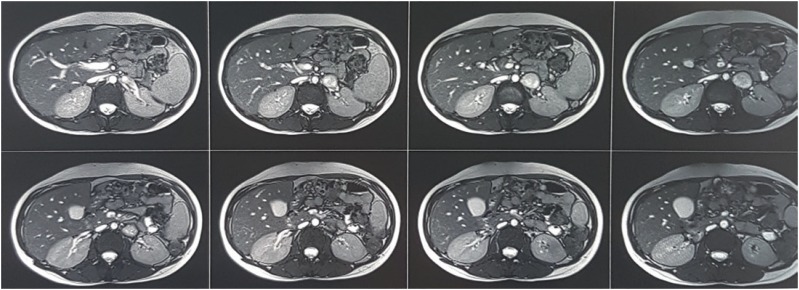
Magnetic Resonance Imaging (MRI) of abdomen showed a round soft tissue mass measuring 3×3.5cm medial to left renal hilum, compatible with extra adrenal pheochromocytoma (paraganglioma).
